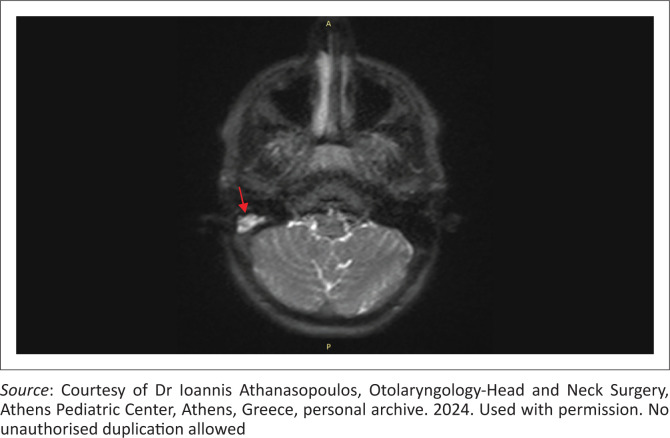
Axial non-echo-planar diffusion-weighted MRI of a 15-year-old male patient, confirming restricted diffusion of keratin debris within the cholesteatoma (red arrow), improving specificity in cases where CT alone is insufficient.
